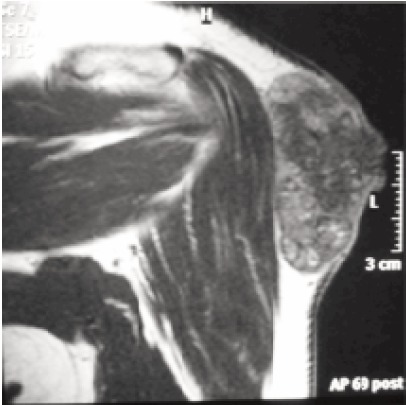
MRI of left shoulder, coronal view shows well defined
